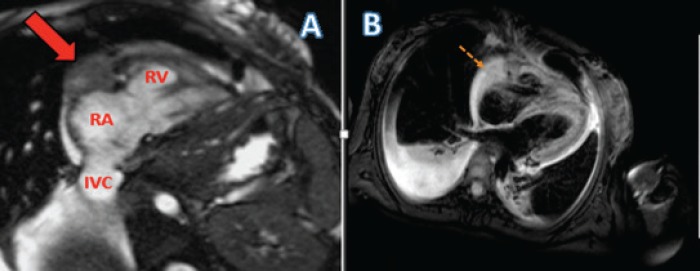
(a) Cardiac MRI of the patient with cine RV-2 chamber view showing the pericardial mass encasing the right atrioventricular groove (AV) groove (solid red arrow). Labelled RA: Right atrium; RV: Right ventricle; IVC: Inferior vena cava. (b) Tissue characteristics of the right atrial mass.
